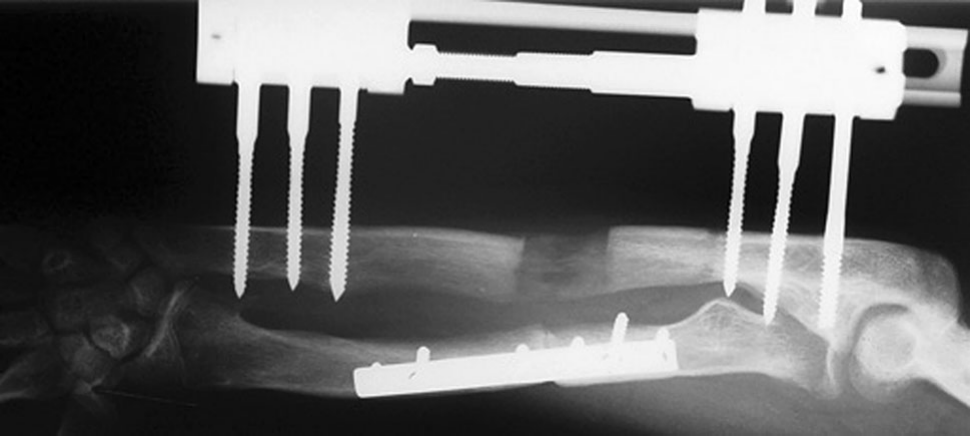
X-ray at the end of lengthening
